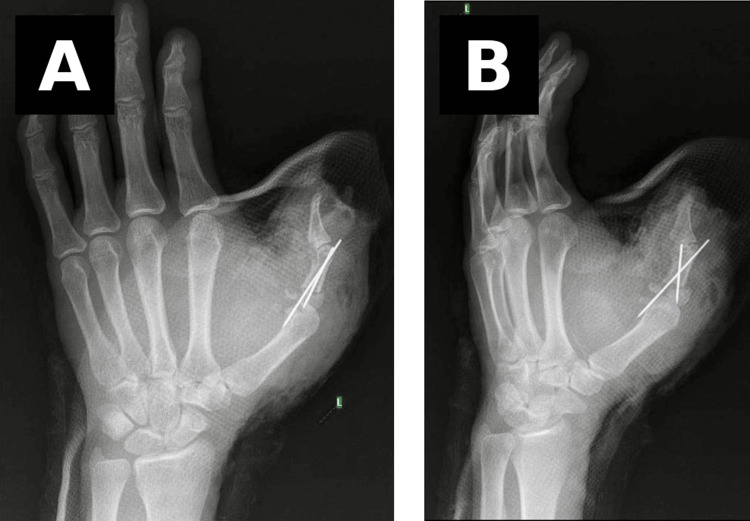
Postoperative radiographs following thumb replantation (A) Anteroposterior view demonstrating K-wire fixation of the proximal phalanx. (B) Lateral view confirming alignment and stability of the replanted thumb.
